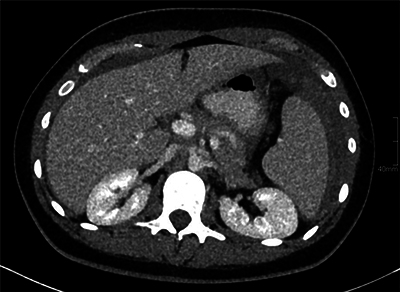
Contrast-enhanced abdominal CT shows a haemoperitoneum. A hyperdense, perisplenic collection with layering suggests an acute perisplenic haematoma.
